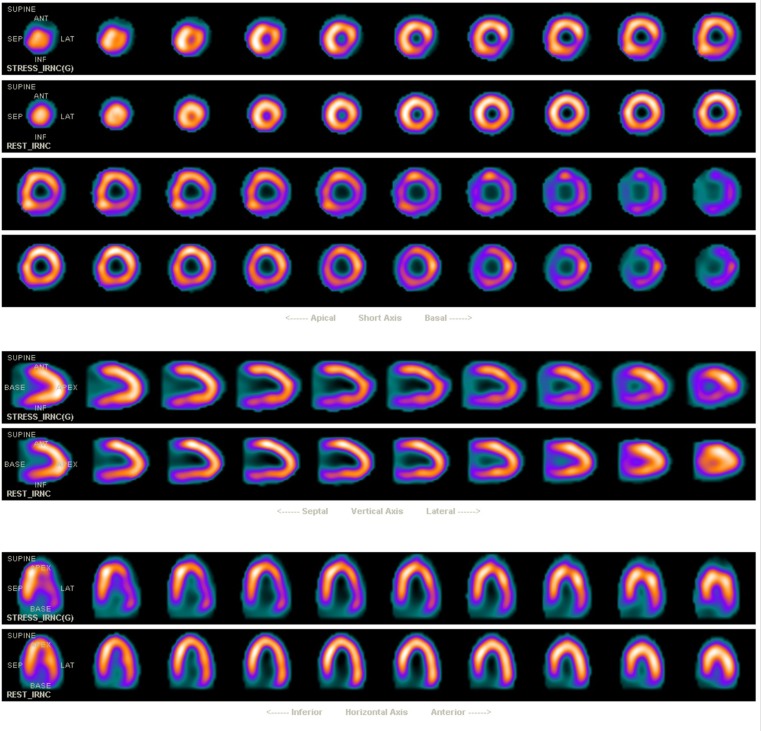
Myocardial perfusion single-photon emission computed tomography without attenuation correction. A reversible moderate size lateral perfusion defect is demonstrated
